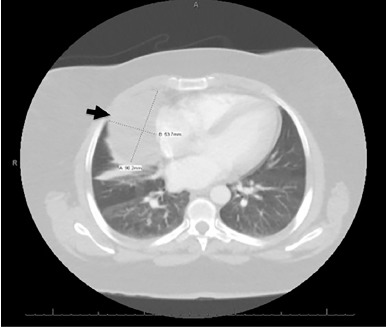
Axial slice of computed tomography (CT) of the chest (pulmonary embolism protocol): CT showing pericardial cyst (arrow).
